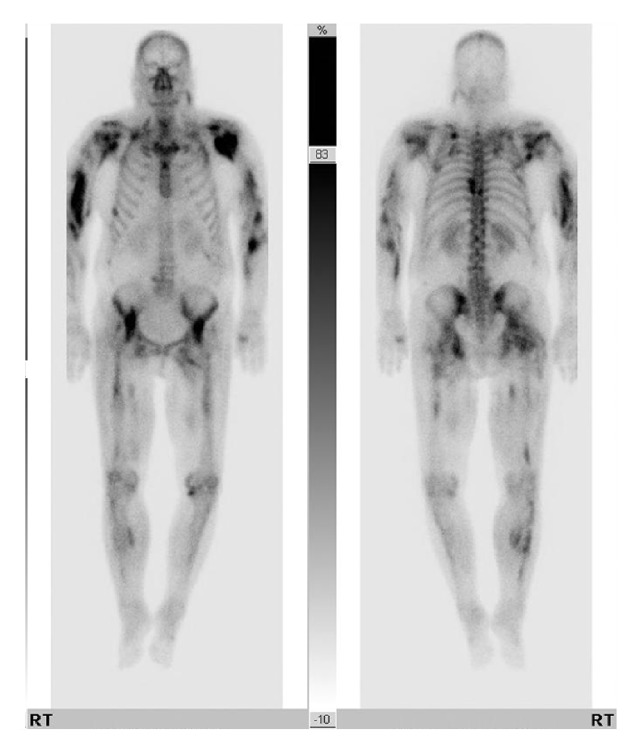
Whole body bone scintigraphy shows radioiodine uptake in multiple locations. Whole body bone scintigraphy shows radioiodine uptake in the head, shoulder, hip, and both upper and lower extremities, suggesting rhabdomyolysis.
